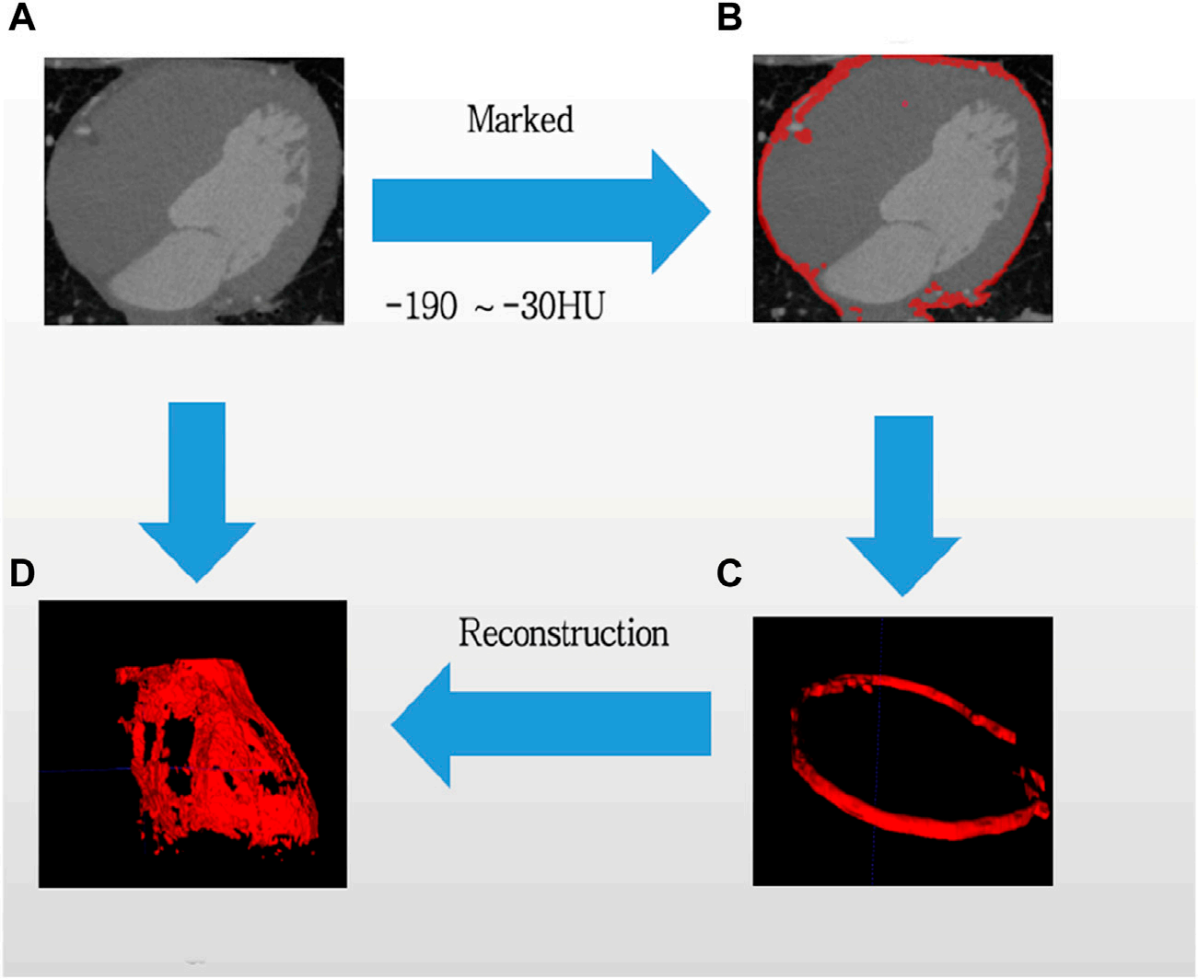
Computed tomography is utilized to evaluate epicardial adipose tissue. From original CT (A), then selected the threshold value from −190HU to −30HU, and Marked the EAT tissue (B), updated a flat EAT tissue (C), finally reconstructed the images of EAT. Adipose tissue was emphasized in red, while the process was indicated by blue arrows. (D) Is reconstruction of EAT.
